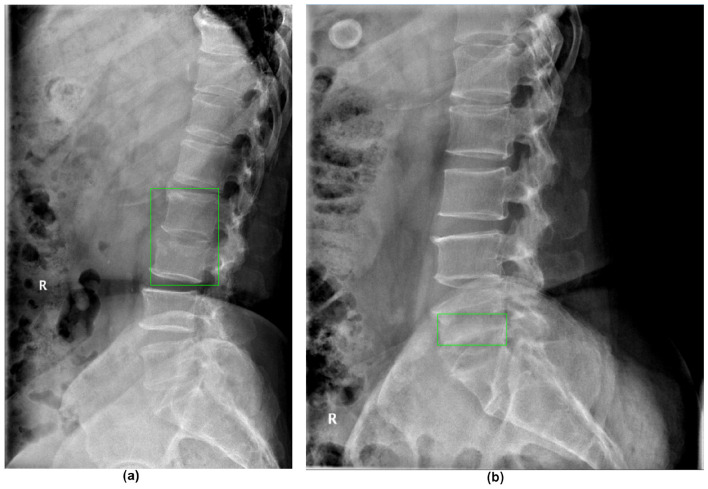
Examples of suspicious-region prompts generated by the YOLOv11 model in two spinal tuberculosis cases with equivocal X-ray findings. (a) Example showing a suspicious region spanning multiple adjacent vertebral levels. (b) Example showing a more localized suspicious region. Green boxes indicate the suspicious regions predicted by the YOLOv11 model.
